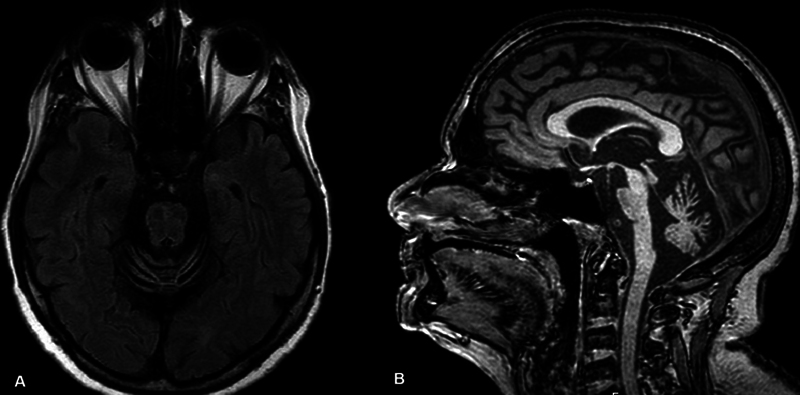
Brain magnetic resonance imaging of a patient with multiple system atrophy – cerebellar form: (
